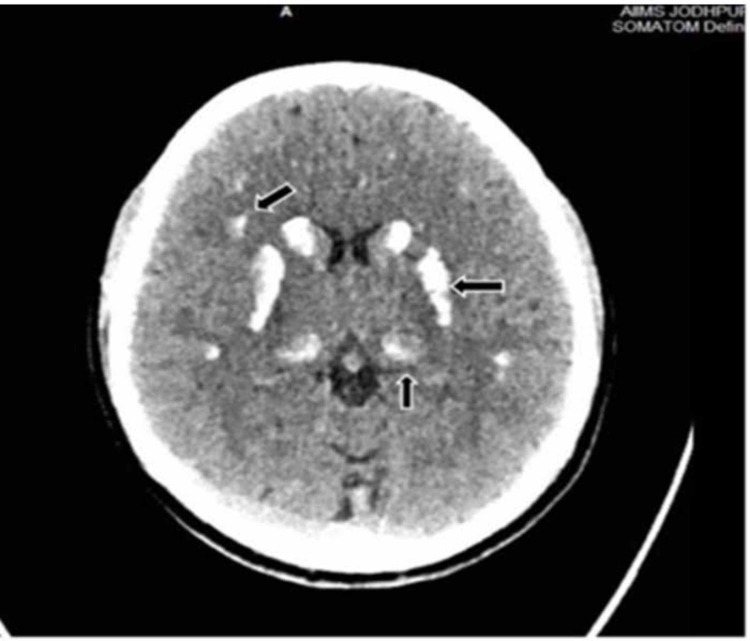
Non-contrast computed tomography Head showing large foci of parenchymal calcifications seen in bilateral putamen, thalami and grey-white matter junctions in bilateral frontal and parietal lobes. (Arrowed- Right frontal, left putamen and thalamus)
